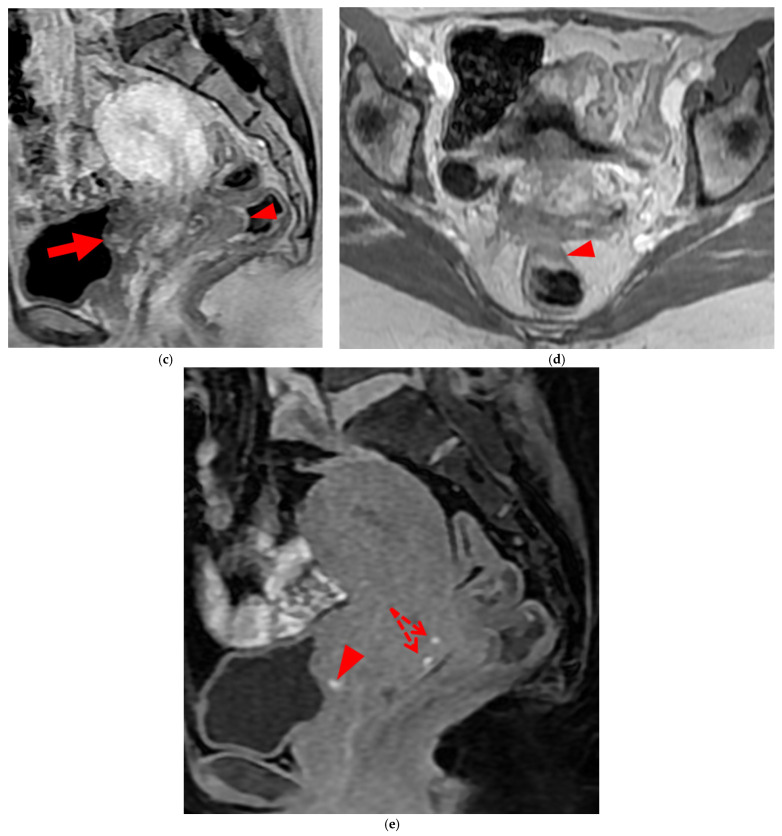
Pelvic MRI scan of a patient with HTD type 6 USLs. (a–d) Sagittal (a) and axial (b) T2WI, sagittal (c) and axial (d) contrast-enhanced T1WI demonstrate involvement of the posterior bladder wall (*arrows*) and rectal wall infiltration appearing as a “medallion-shaped” lesion (*arrowheads*). (e) Sagittal fat-suppressed T1WI shows two hyperintense hemorrhagic spots at the origin of the USLs (*dashed arrows*) and another within the bladder lesion (*arrowhead*). The presence of “visceral” involvement of both the digestive and urinary tracts results in reclassifying these type 4 USLs as type 6.
